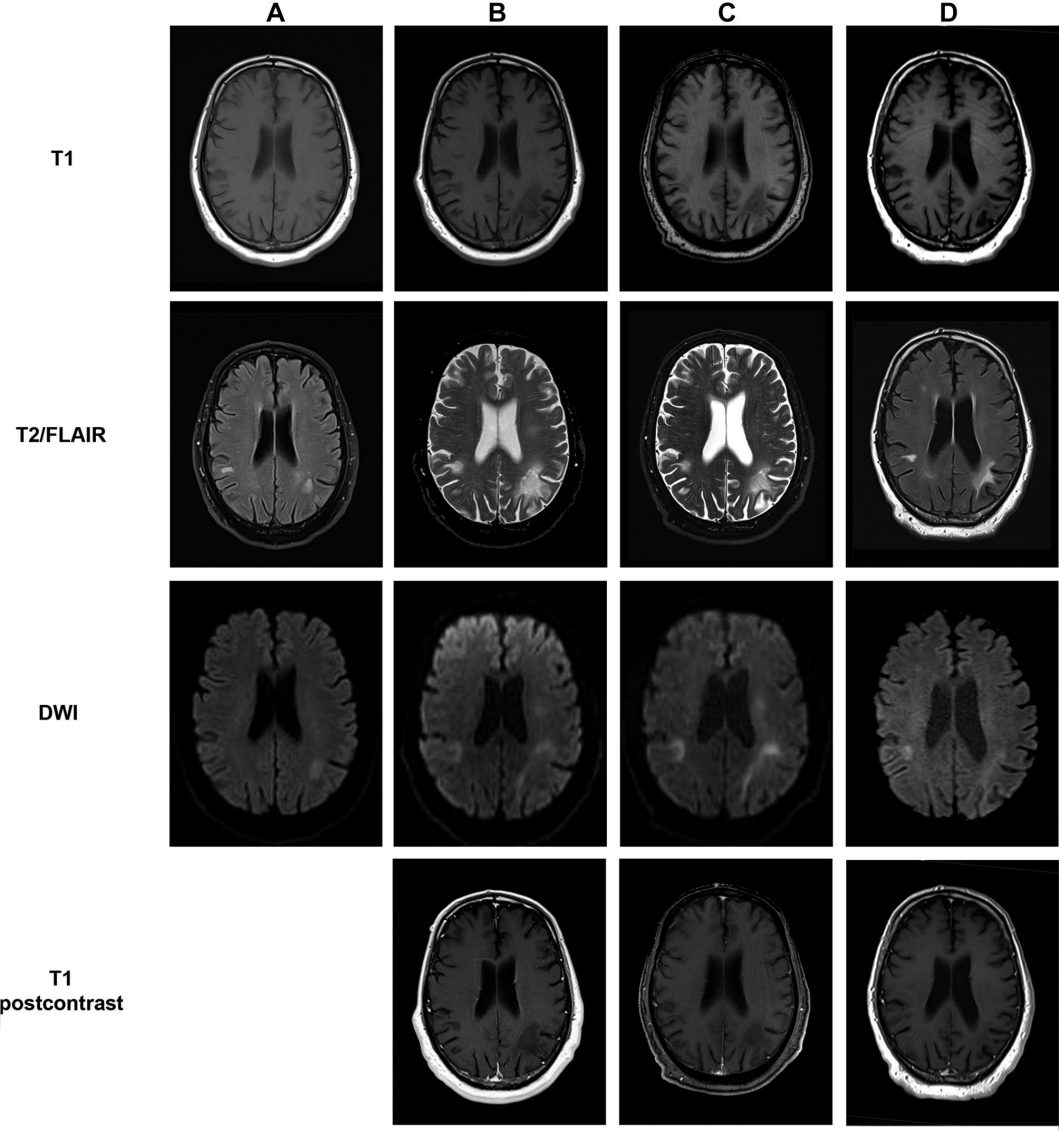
MRI of the head during treatment with pembrolizumab. Shown is a panel with sequential MRI scans of the head from July 2019 (A), before the third pembrolizumab infusion in January 2020 (B), the fifth pembrolizumab infusion in February 2020 (C), and after the final treatment with pembrolizumab in a follow-up examination in August 2020 (D). Shown sequences are T1 (A–D), T2-weighed (B–C) or FLAIR (A, D), DWI (A–D), and T1 postcontrast (B–C)
